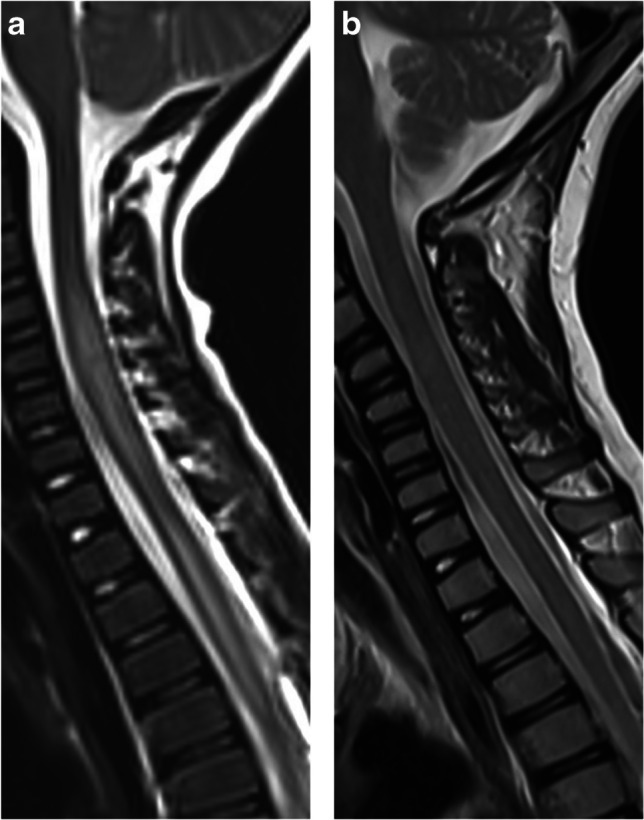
Lesion dynamics in a 4-year-old male with myelin oligodendrocyte glycoprotein antibody-associated disease (MOGAD). a, b Sagittal T2-weighted magnetic resonance images obtained from a spinal scan during attack (a) and at 1-month follow-up (b) show typical MOGAD interval complete resolution of the abnormal T2-hyperintensity along the cervical and upper thoracic cord
